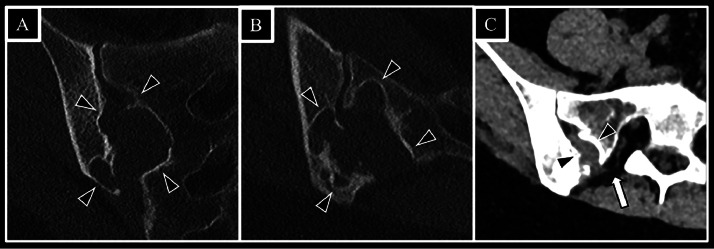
Incidental finding of a right sided sacro-iliac mass on CT. (A) Coronal oblique reconstruction, bone window; (B) axial oblique reconstruction, bone window; (C) axial reconstruction, soft tissue window; black arrowheads exhibit the meningioma, white arrow exhibit close contact with the dorsal ramus of S1.
